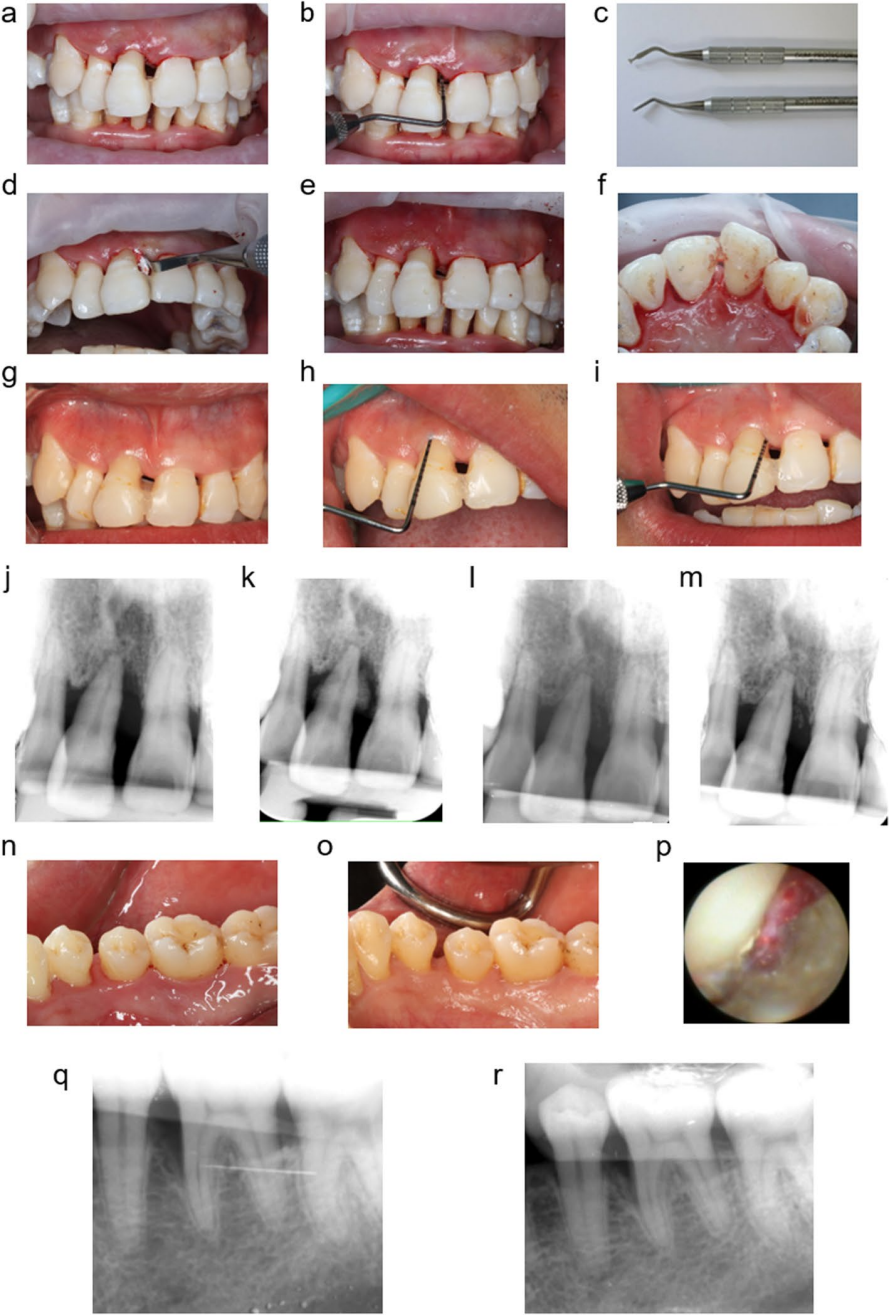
Typical cases treated by periodontal endoscopy-aided non-incisional periodontal regeneration technique (NIT) or periodontal endoscopy-aided scaling and root planing (PSRP). a-m a severe case treated with NIT. a Clinical image of buccal side of the right maxillary central incisors. b 10 mm probing depth (PD) was detected at buccal side. c Gingival retractor. d The placement of bone substitute (Bio-Oss collagen). e-f Clinical image of buccal and palatal side after the placement of bone substitute. g-i Clinical image of buccal side at 1-year evaluation after NIT. 2-4 mm PD was measured. j Baseline radiograph. k Periapical radiograph immediately after NIT. l Periapical radiograph at 3 months after the treatment. m Periapical radiograph at 1-year after the treatment. n-r a case treated with PSRP. n Clinical image of the left mandibular first molar at baseline. o Clinical image of the left mandibular first molar at 1-year after the treatment. p Subgingival field under the periodontal endoscope. q Baseline radiograph. r Periapical radiograph at 1-year after PSRP
